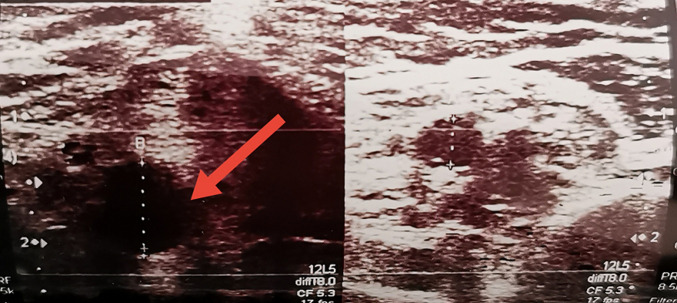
échodoppler; artère non compressible
